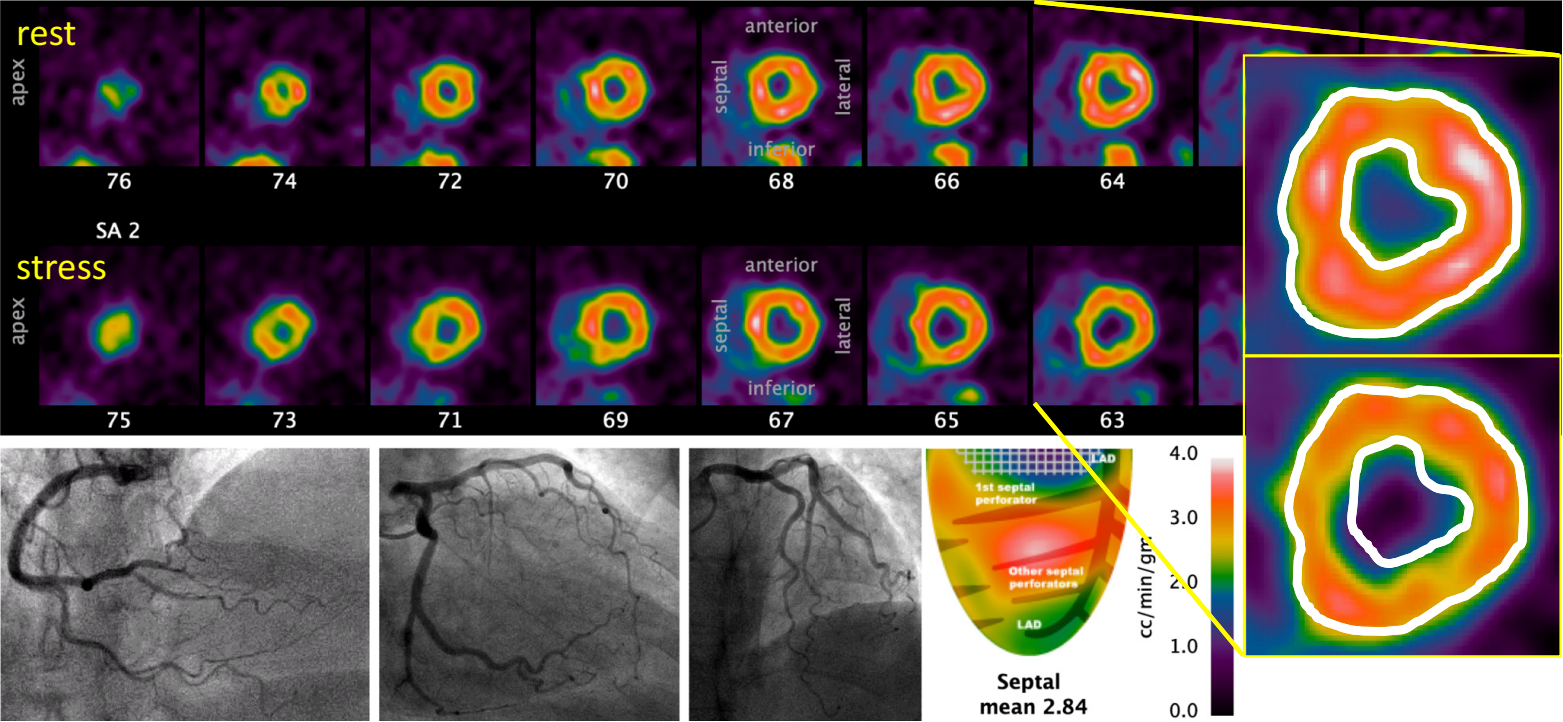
Subendocardial angina with non-obstructive epicardial arteries*. This 68-year-old woman suffered from exertional angina and dyspnea for several years, prompting an invasive angiogram that demonstrated at most mild atherosclerosis. Echocardiography demonstrated normal left ventricular (LV) function and LV hypertrophy but no significant valvular disease. Due to persistent symptoms, she underwent a cardiac PET scan with dipyridamole stress during which she developed severe angina. An excellent average stress flow of over 2.8 cc/min/g excludes microvascular dysfunction. While no regional defect is present, the inset images copy the resting epicardial and endocardial borders onto the stress tomographic images. A nearly circumferential subendocardial perfusion defect explains her symptoms and would not have been identified by epicardial assessment of FFR, flow reserve, or myocardial resistance
